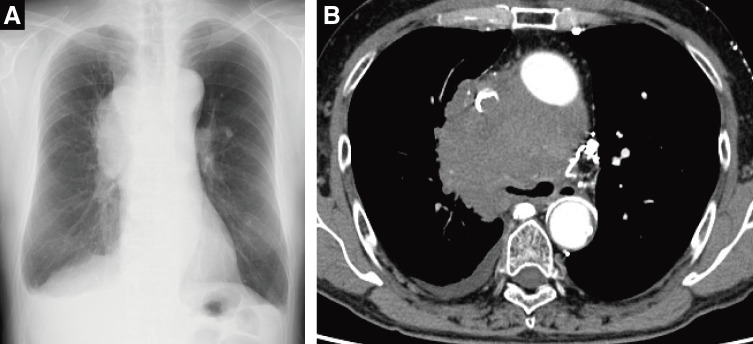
Radiological imaging before treatment. (A) A chest radiograph revealed enlargement of the mediastinum and a tumor in the right hilum. (B) Contrast-enhanced CT revealed an 11-cm tumor in the mediastinum, compressing the trachea and superior vena cava. CT, computed tomography
